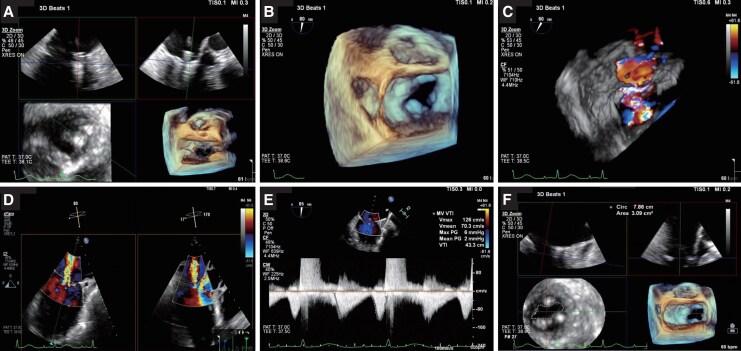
First clip implantation under transoesophageal echocardiographic guidance (*A–B*) deployment of the first clip at the lateral commissural region. (*C–D*) Residual central mitral regurgitation after first clip implantation. (*E*) Mean transmitral pressure gradient after first clip implantation. (*F*) Mitral valve area after first clip implantation.
